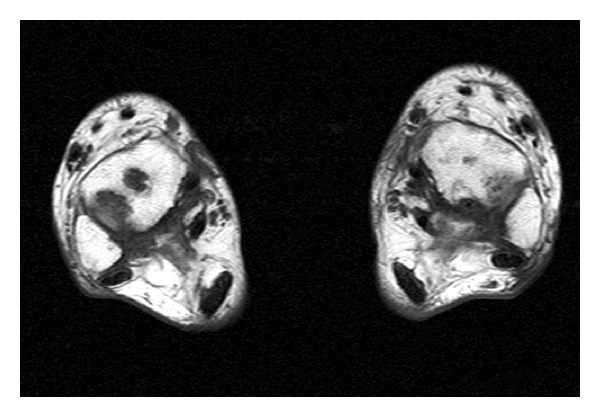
MRI TSE T1 axial view of the ankles at the level of the talar dome.
